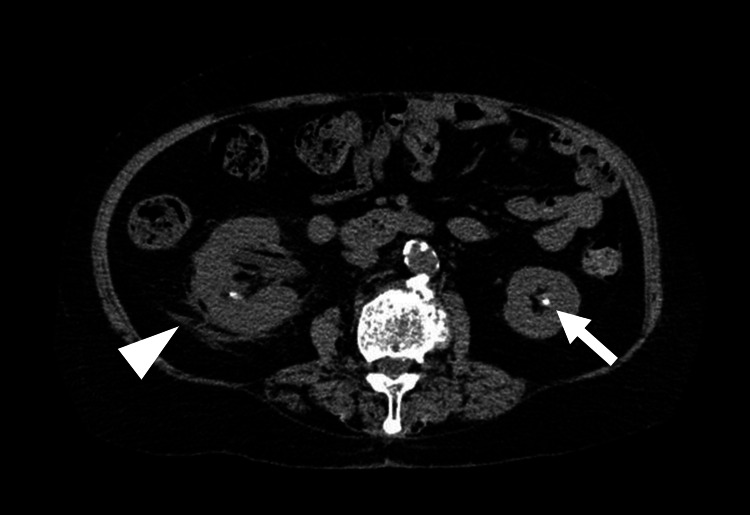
Abdominal computed tomography (CT) on admission CT showing right hydronephrosis, kidney swelling, a right kidney stone, and perirenal fat stranding (arrowhead). It also shows a left kidney stone (arrow).
